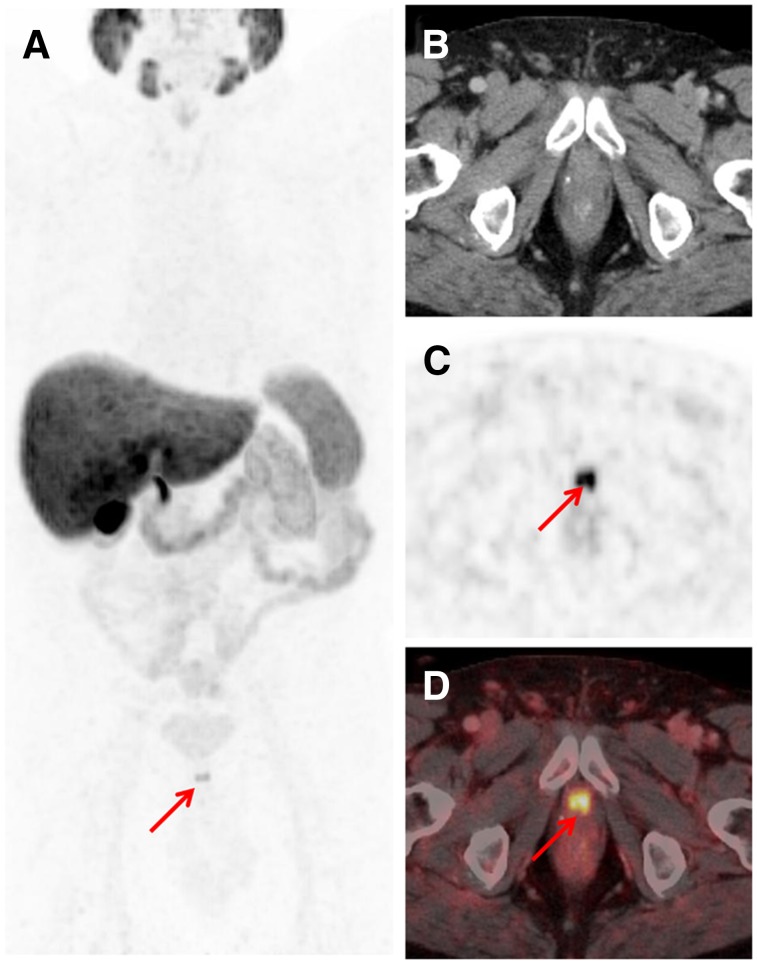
Images from 64-y-old patient after radical prostatectomy (August 2012; Gleason score of 9; pT3b; pN0) and with PSA level rising to 3.9 ng/mL (October 2017). (A) Maximum-intensity projection of ^18^F-PSMA-1007 PET shows intense tracer-associated uptake in lesion (arrow) below bladder. (C and D) It could be localized (arrows) in region of urethral anastomosis using transaxial PET (C) and fused PET/CT (D) images. (B) Corresponding CT image. Postimaging salvage radiation to prostatic fossa was performed in combination with single injection of gonadotropin-releasing hormone analog in October 2017 and resulted in drop in PSA level to below detection threshold (<0.07 ng/mL; last measurement in February 2018).
